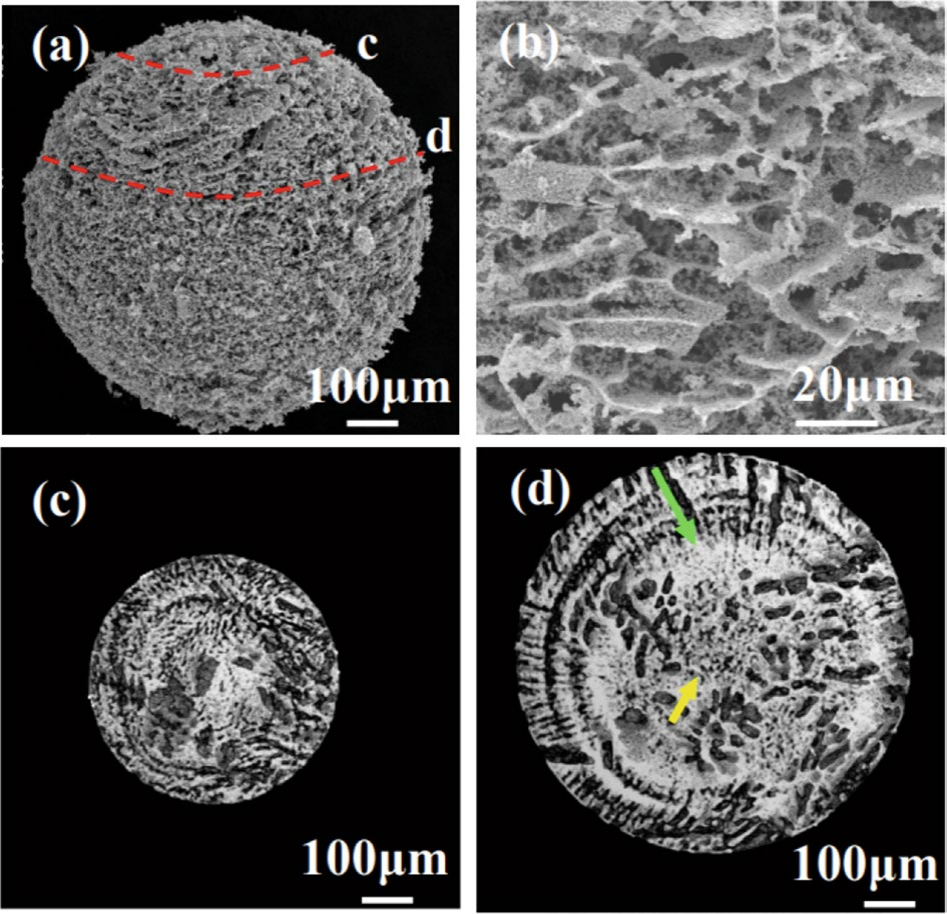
(a) low-magnification and (b) high-magnification SEM images of a Mo-30at%Si FD-POEM particle. (c,d) The X-ray CT images of the areas marked by red lines in (a).
